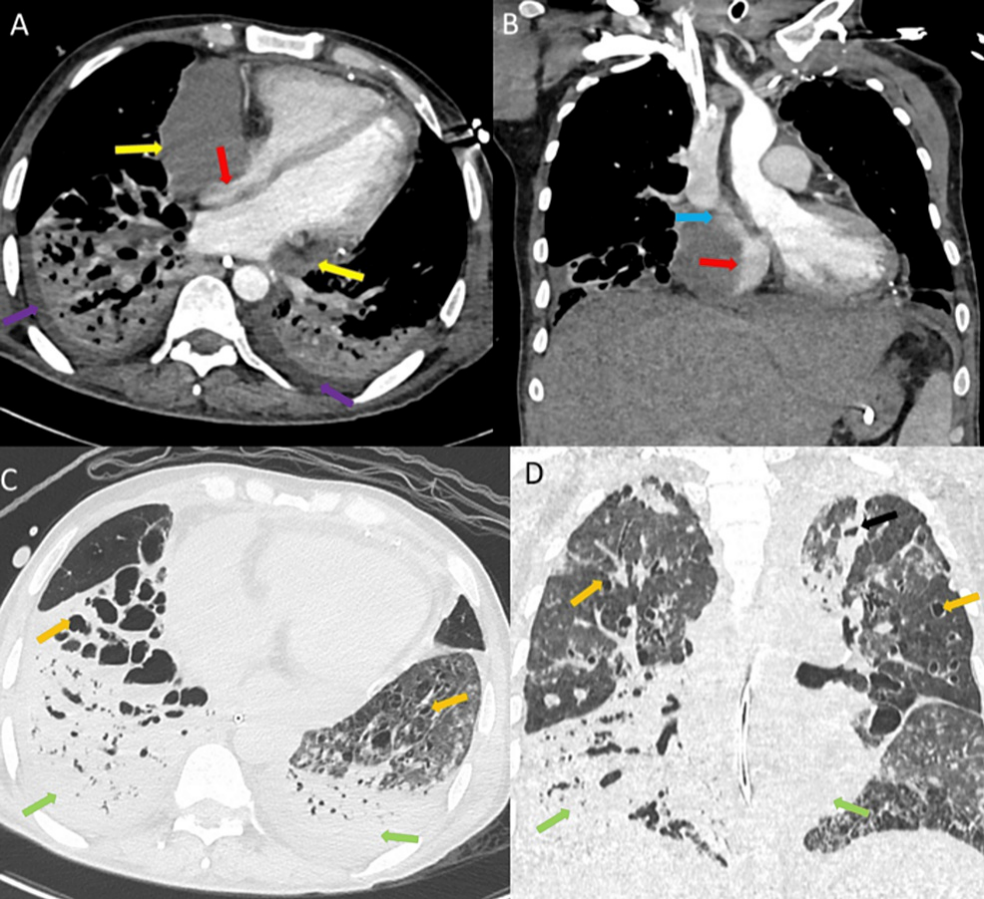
CT of the thorax with IV contrast, soft tissue window A) Axial and B) coronal cuts showing significant large pericardial effusion involving the inferior and right pericardial regions (yellow arrows) causing severe compression on the right atrium with almost total obliteration of the lumen (red arrow) and compression on the lower end of the superior vena cava (blue arrow). Lung window C) axial and D) coronal images demonstrating bilateral areas of consolidation, ground glass opacification, and centrilobular nodules in both upper lobes with consolidation in the lower lobes (green arrows); bilateral cystic bronchiectasis (orange arrows), left upper lobe irregular cavity (black arrow), and newly formed bilateral pleural effusion (purple arrows) were also seen CT: computed tomography
